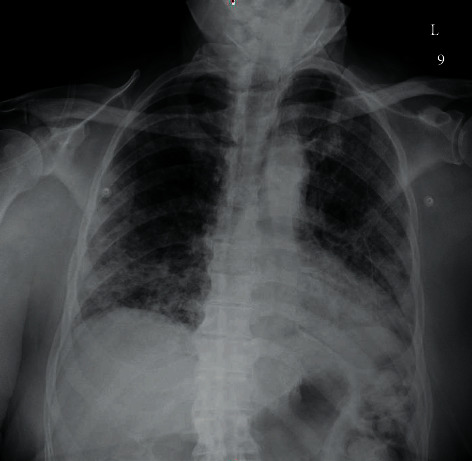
Chest X-ray anteroposterior on admission: moderately extensive bilateral patchy airspace disease.
